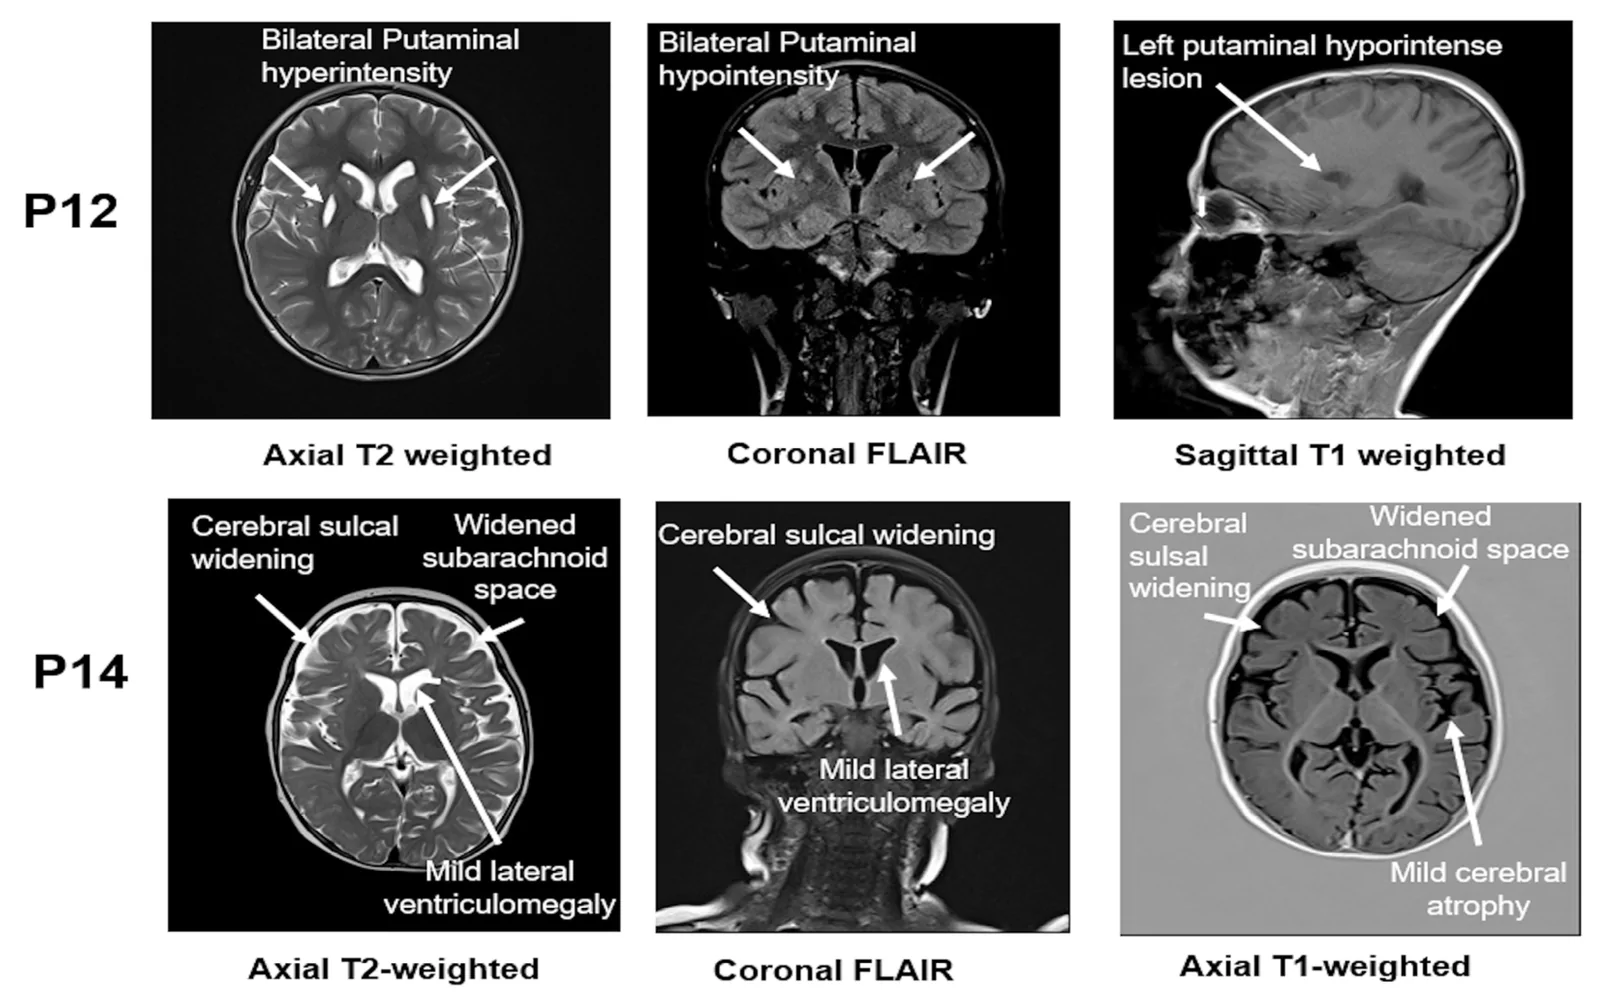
Magnetic resonance imaging of the brain in patients P12 and P14. P12: symmetric hyperintense lesions in bilateral putamen on an axial T2-weighted image; symmetric hypointense lesions in the bilateral putamen with a hyperintense rim on a coronal fluid-attenuated inversion recovery (FLAIR) image; and a hypointense lesion in the left putamen in a sagittal T1-weighted image. P14: widening of the cerebral sulci and subarachnoid space in the supratentorium, associated with mild dilatation of the lateral ventricles on an axial T2-weighted image; widening of the cerebral sulci and the subarachnoid space in the supratentorium, associated with mild dilatation of the lateral ventricles on a coronal FLAIR image; and widening of the cerebral sulci and subarachnoid space in the supratentorium, associated with mild dilatation of the lateral ventricles and consistent with mild cerebral atrophy on an axial T1-weighted image.
